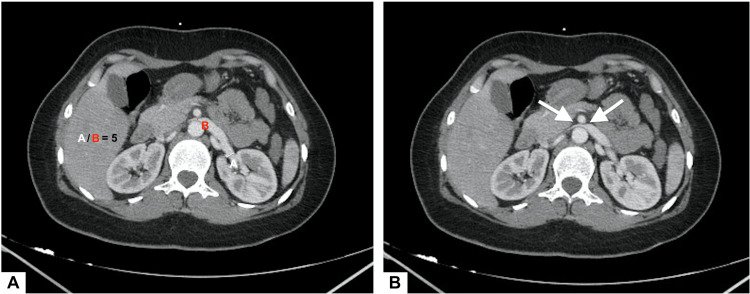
Axial CT angiography showing the compressive phenomenon A: Dilatation of the left renal vein with a ratio of hilar to aorto-mesenteric diameters > 4,9 (A/B = 5). B: White arrows indicating the narrowing of left renal vein segment trapped between the abdominal aorta and the superior mesenteric artery ("beak sign")
